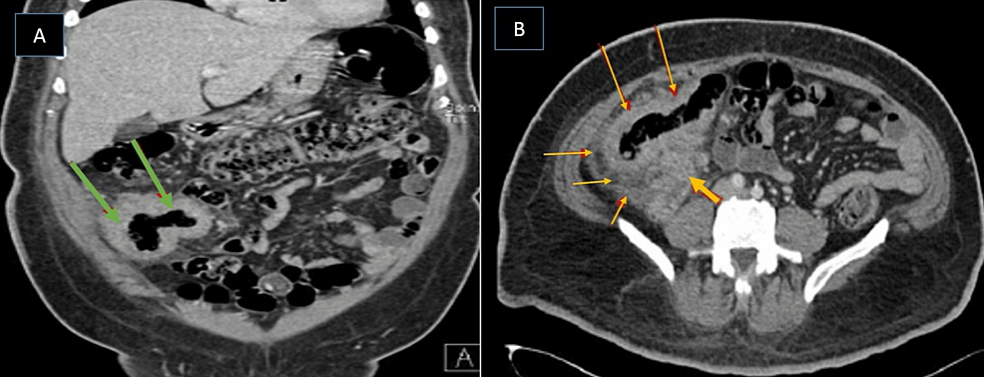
Enhanced CT scan of the abdomen and pelvis (Case 5) A and B: Enhanced CT scan of the abdomen and pelvis (A: sagittal plane, B: axial plane) shows marked irregular thickening of the terminal ileum and cecum (long arrows) with pericolonic inflammatory fat stranding (orange arrows) and subcentimeter lymph nodes. The appendix (green arrows) is diffusely thickened with heterogeneous enhancement as well.
